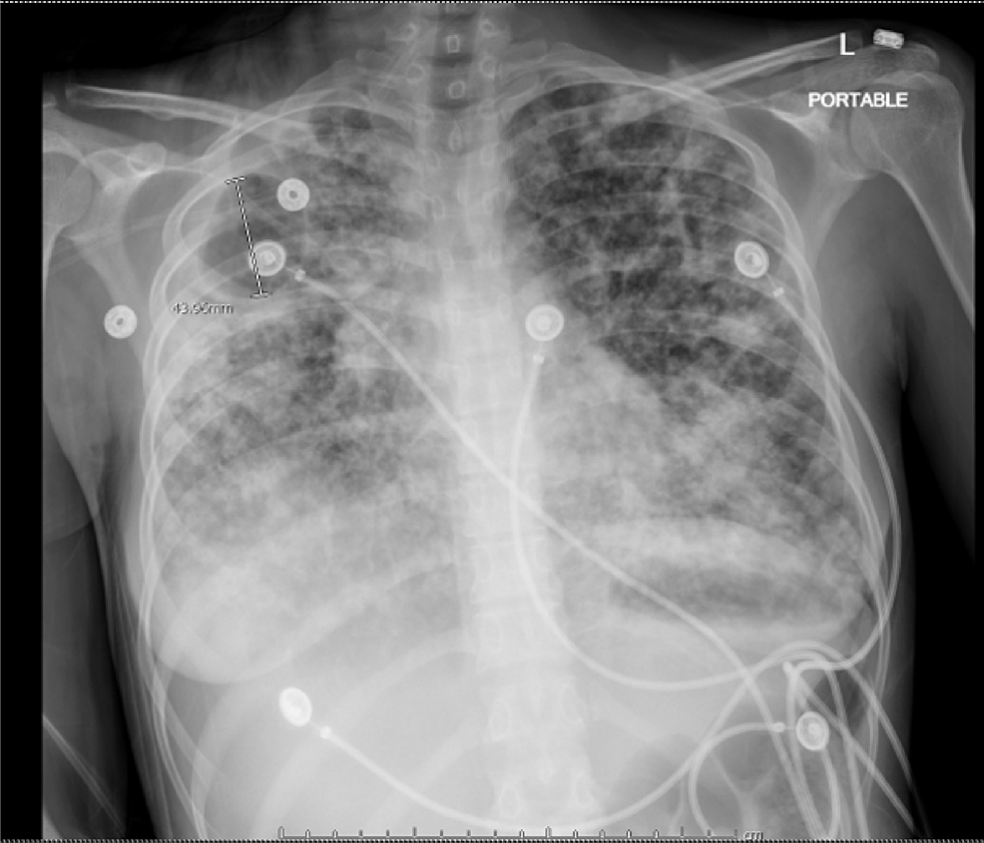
Chest radiograph revealing diffuse infiltrates with apical right cavitary lesion
